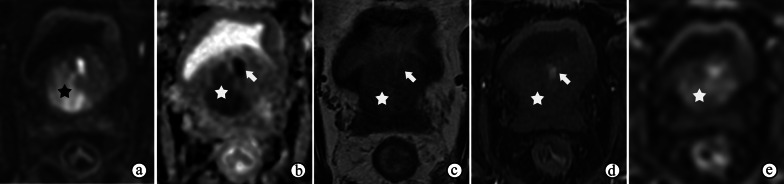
Intraductal carcinoma of the prostate in an 80-year-old man, with an elevated serum PSA level for 2 years, which was 24.46 ng/mL at the most recent measurement. a, b DWI at a b-value of 1400 s/mm^2^ and ADC maps show a lobulated mass with restricted diffusion in the prostate (☆). c Axial T2-weighted imaging shows isointensity with an ill-defined edge of the mass (☆). d, e Axial DCE MRI at 60 s (d) and 80 s (e) after contrast injection shows no prominent enhancement of the mass (☆). Note the hemorrhagic area in the prostate gland (arrow)
